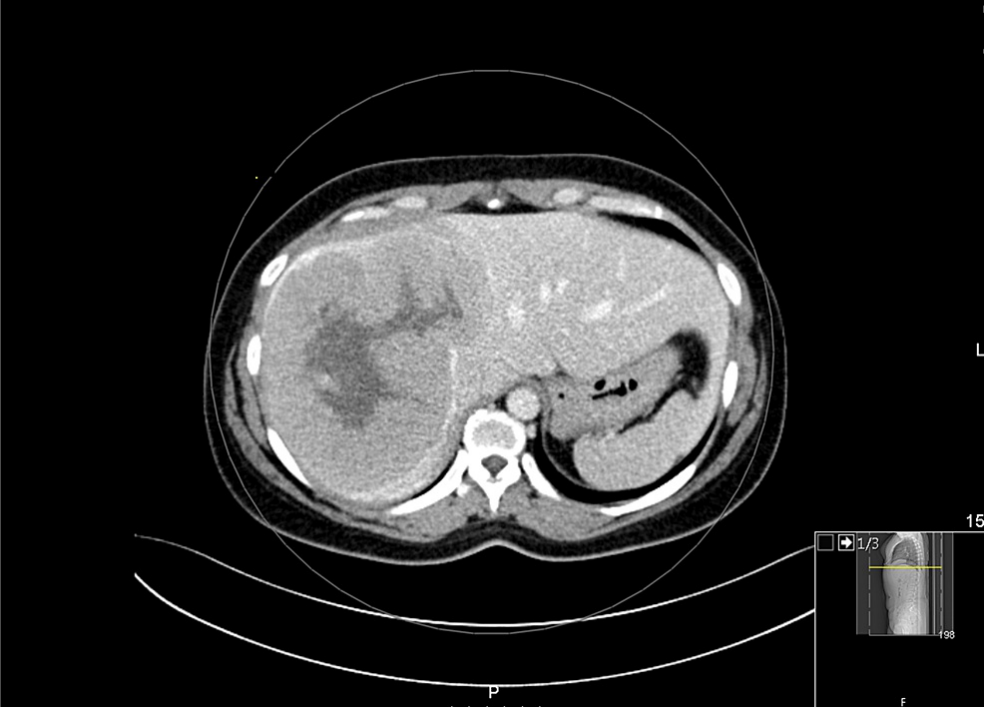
Abdominal CT scan showing a heterogeneous lesion, centered in the right lobe of the liver, measuring 12x16 cm, with hypodense central areas, suggesting some necrotic component.
